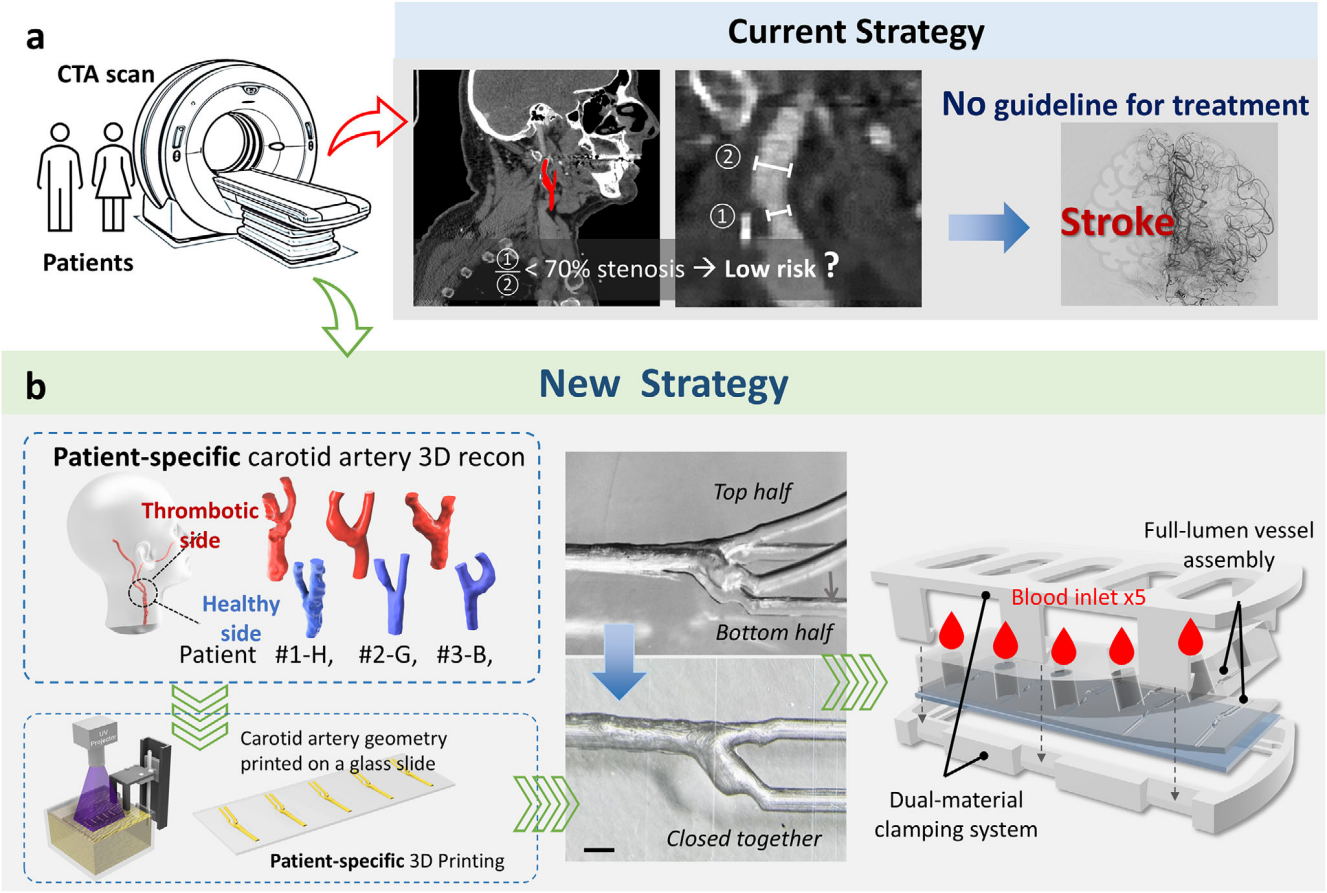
Microprecision 3D fabrication of patient‐specific Carotid Artery‐Chip for personalized thrombotic assessment. a) The current strategy to assess stroke risk relies on measuring carotid artery stenosis. *Left*: Patients undergo CTA imaging of carotid arteries to diagnose the cause of stroke and determine treatment strategies. *Middle*: CTA images are used to measure stenosis levels, with stenosis defined as the ratio of the narrowed vessel diameter (①) to the downstream normal vessel diameter (②). *Right*: A digital subtraction angiography (DSA) image from an example patient shows less than 70% stenosis with no guideline for treatment, yet the patient eventually developed a stroke. b) Clinical 3D reconstruction of carotid arteries for stroke patients. *Left*: CTA images are utilized to reconstruct patient‐specific carotid artery geometries, including both thrombotic and healthy sides, identified by a number and a letter. *Middle*: The alignment and assembly of the two halves of the patient‐specific Carotid Artery‐Chip. Scale bars = 200 µm. *Right*: The final chip design for personalized thrombotic risk assessment, using a clamp system to mechanically bond the two halves together. The top half is punched with one inlet (*d* = 6 mm) and one outlet (*d* = 1 mm) for each channel to allow blood perfusion.
